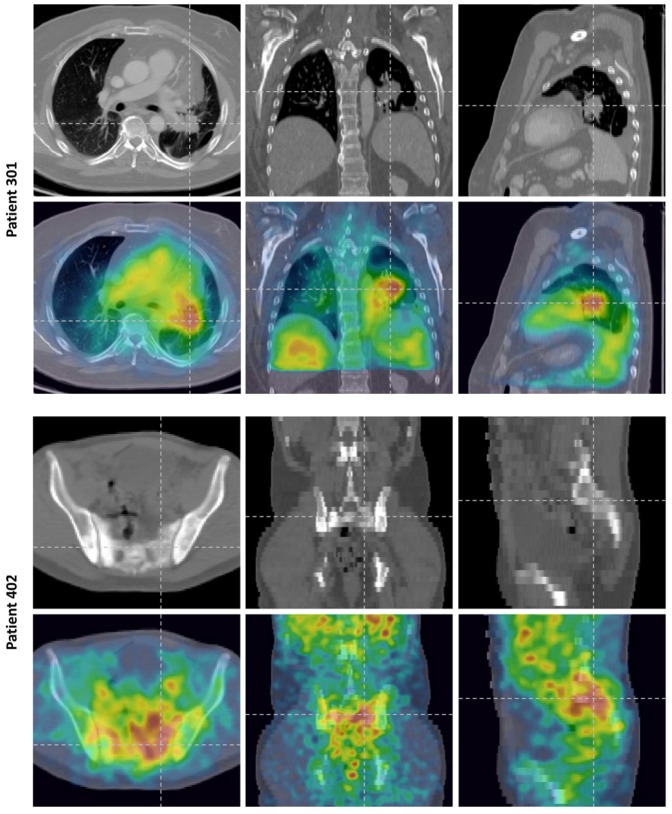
Imaging data from the optional SPECT/CT scans. Top: Day 6 SPECT/CT Images for a subject with colorectal cancer that metastasized to the lung (301). The three columns represent an axial, coronal, and sagittal slice, respectively. The top row shows the diagnostic quality CT alone while the bottom row shows the SPECT data overlayed onto the diagnostic CT. Bottom: Day 6 SPECT/CT Images for a subject with metastatic prostate cancer (402). The top row shows the low dose CT data alone while the bottom row shows the SPECT data overlayed onto the CT data. Note the increased uptake in the osteolytic lesion. The sagittal and coronal slices are of lower resolution compared to the axial plan because the CT was not of diagnostic quality; it was only used for attenuation correction of the SPECT data. A diagnostic quality CT of this subject was unavailable.
